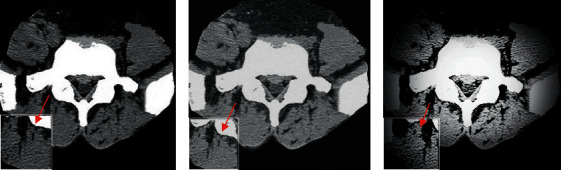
Comparison on CT image segmentation effects of different algorithms. (a) LBF algorithm; (b) PreWork algorithm; (c) AI algorithm.
